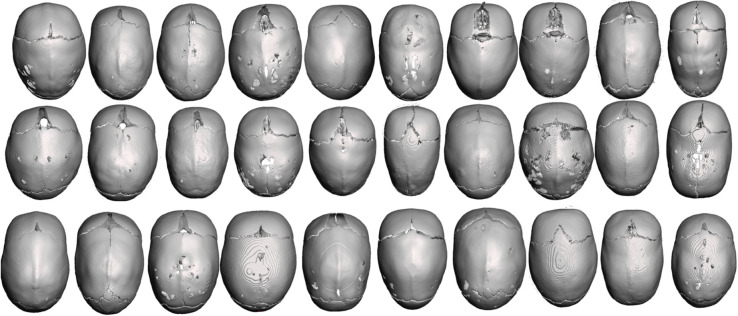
Upper view of the CT scans of the population.
